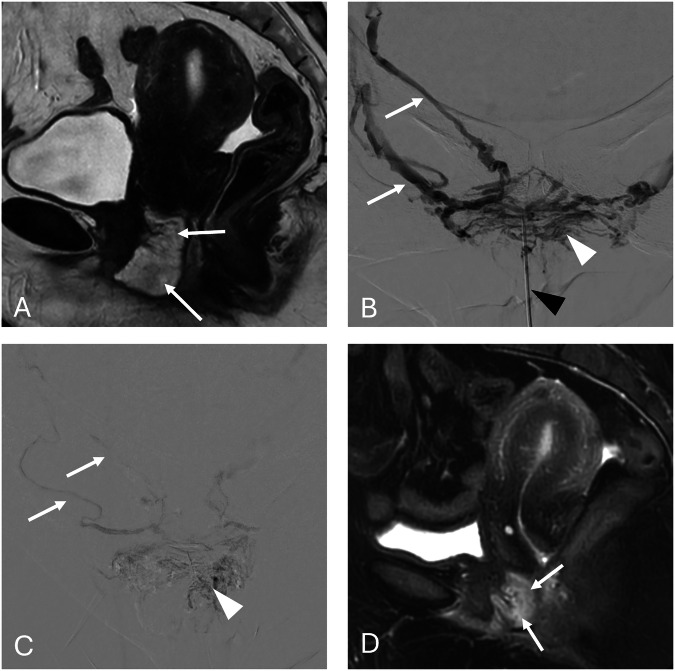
Vaginal gynaecological venous malformation (GVM) treated by transperineal sclerotherapy in a context of severe and persistent vaginal bleeding. A Sagittal T2-W MR image obtained before treatment shows a well-defined, subtly heterogeneous T2-hyperintense lesion of the anterior vaginal wall (arrows), with no visible flow-voids and no involvement of the rest of the genital tract. B Coronal image of transperineal venography before sclerosing agent injection shows dilated dysplastic venous channels of the vaginal GVM (white arrowhead) with several draining veins (arrows), not retrospectively visible on MRI. As a transperineal procedure, a needle was directly inserted within the dysplastic vessels of the lesion (black arrowhead). C Coronal image of transperineal venography after injection of 5 mL of sclerosing agent shows a satisfactory result with minimal to no residual vascular flow in the vaginal GVM (white arrowhead) nor in draining veins (arrows). Sclerosing agent consisted of an emulsion of 2 mL of 3% aetoxisclerol, 0.8 mL of lipiodol, and 2 mL of air. D Sagittal T2-W fat-suppressed MR image obtained at 4-month follow-up shows a significant reduction in lesion size (arrows). The patient reported complete resolution of symptoms
